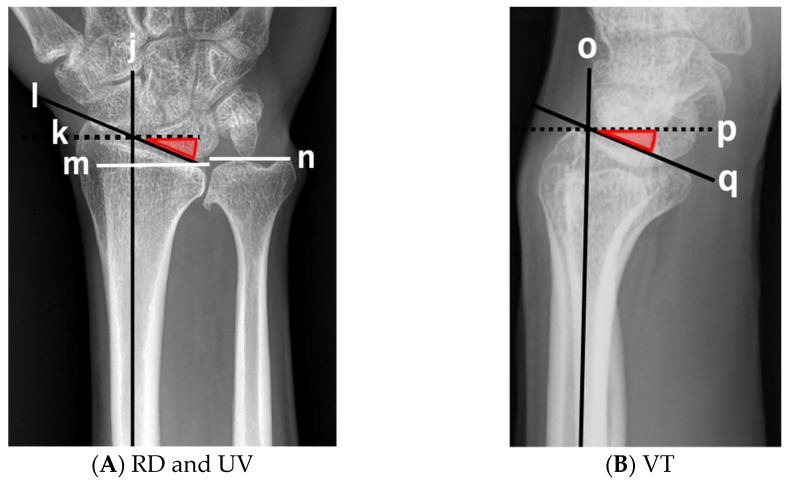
Measurement in the wrist joint. j: long axis of the radius in posteroanterior view, k: line perpendicular to long axis of radius in posteroanterior view, l: tilt of the distal end of the radius on posteroanterior radiograph, m: distal end of the radius, n: distal end of the ulna, o: long axis of the radius in lateral view, p: line perpendicular to long axis of the radius in lateral view, q: tilt of the distal end of the radius on lateral radiograph. (A) Radial deviation (RD) is determined by lines k and l. Ulnar Variance (UV) is the sum of lines m and n. (B) Volar tilt (VT) is determined by lines p and q.
